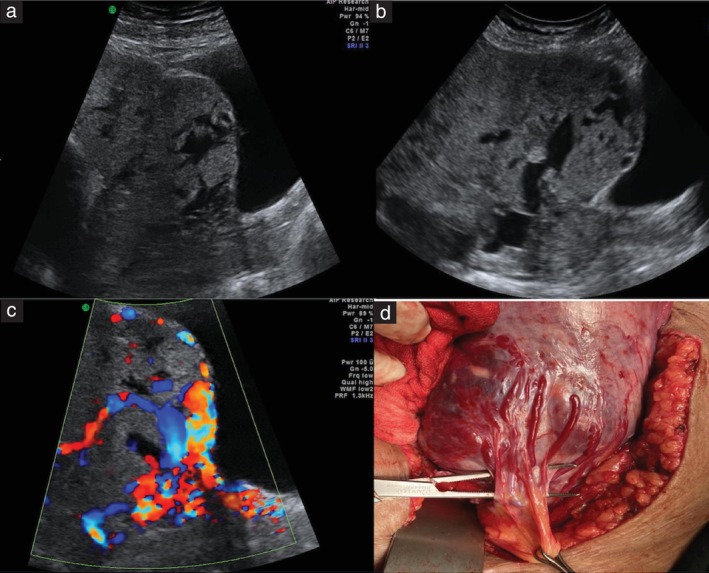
(a,b) Grayscale ultrasound images in a patient with two previous Cesarean deliveries and anterior placenta previa with features of extensive lower uterine segment remodeling (placental bulge, myometrial thinning and loss of clear zone). (c) On color Doppler imaging, features of uteroplacental vascular remodeling (abnormal lacunae, lacunae feeding vessel and uterovesical hypervascularity) as well as serosal hypervascularity (bridging vessels and bladder wall interruption) were observed. (d) The intraoperative image shows a placental bulge and numerous vessels on the serosal surface, with anastomosis to the vesical vessels. A diagnosis of high‐grade placenta accreta spectrum was made based on the intraoperative appearance and the failure of placental separation.
